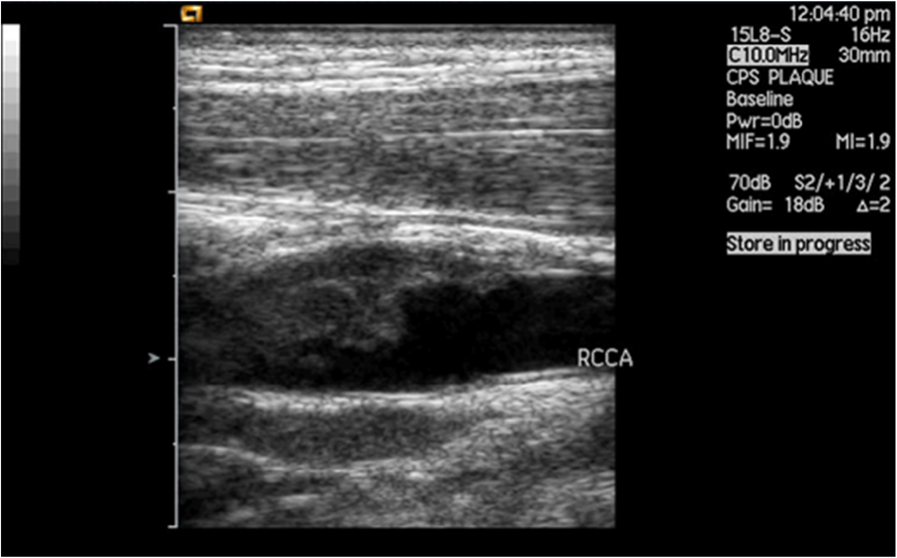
Carotid ultrasonography showed that the diameters of bilateral common carotid artery were normal. A hypoechoic mass with irregular contour was observed at the right common carotid artery. The size of the mass was about 29 × 7mm, with heteroechogenicity.
